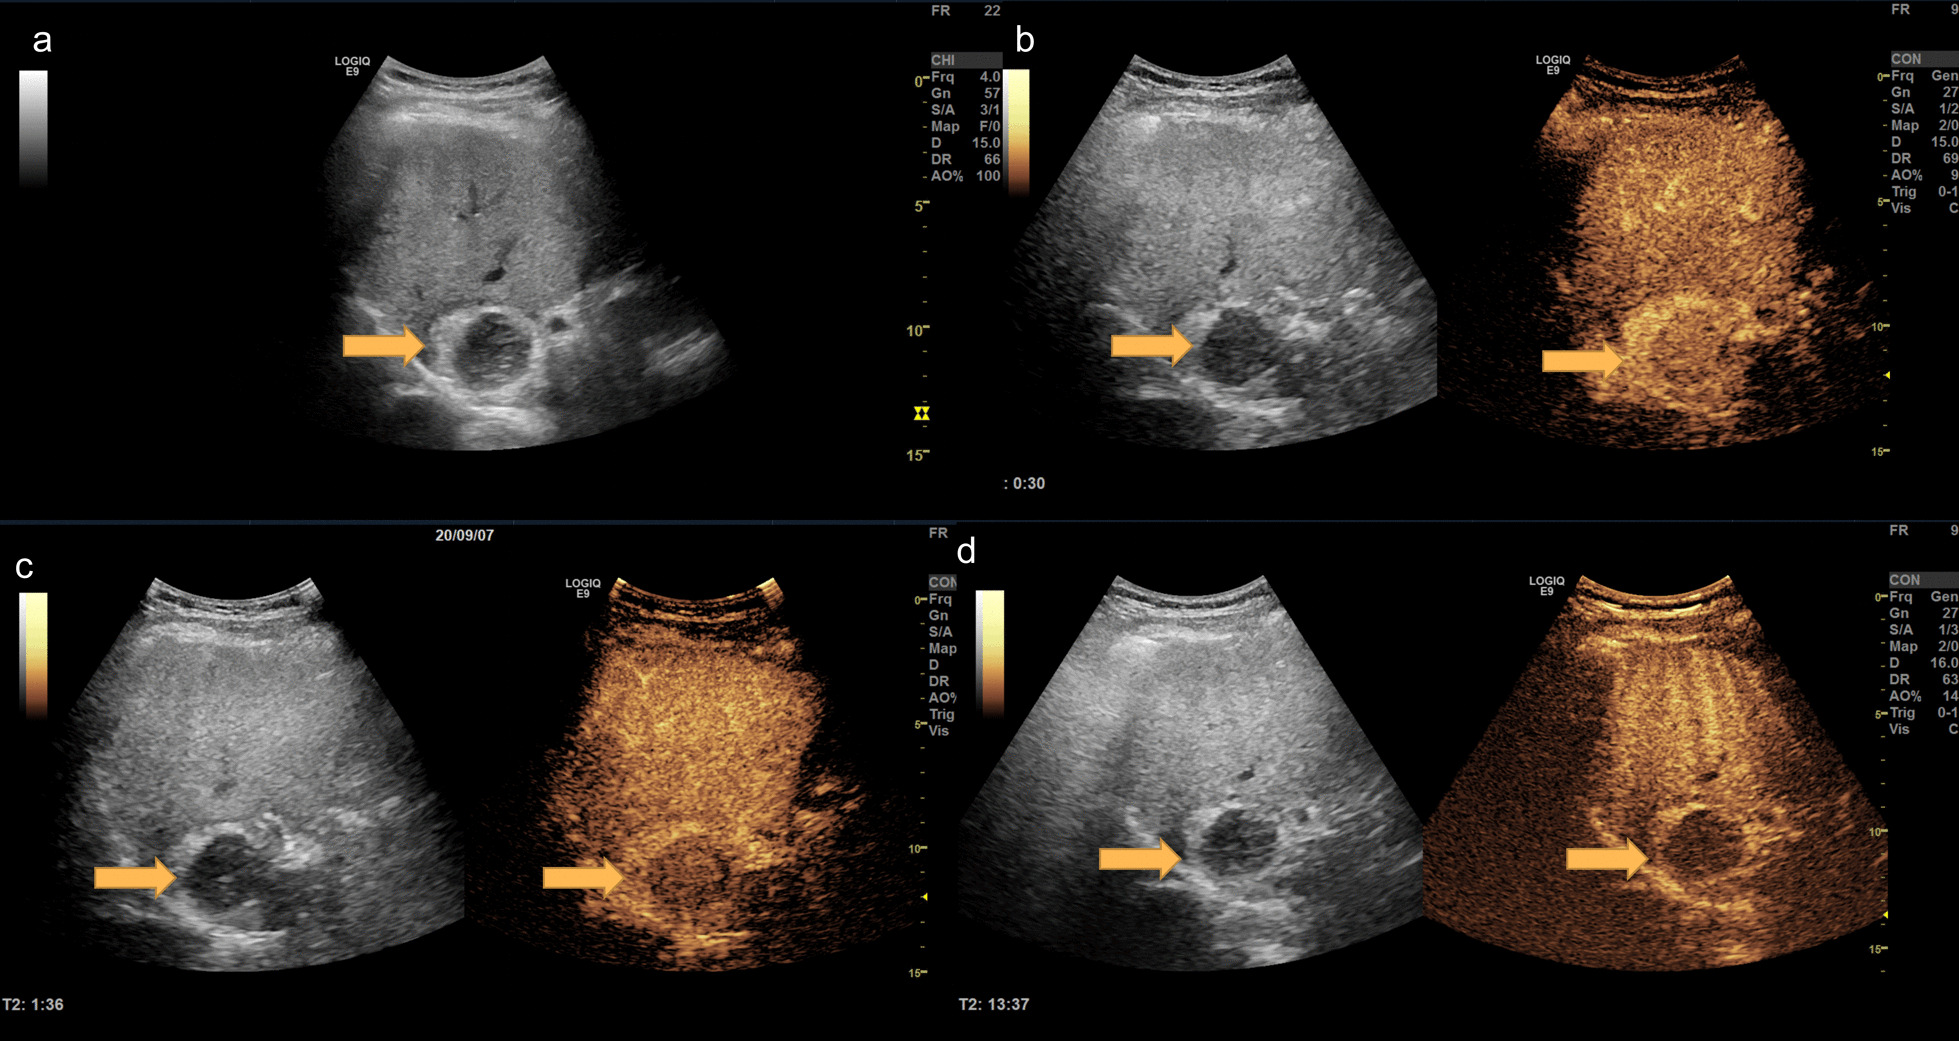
A liver mass discovered during physical examination in a 30-year-old male patient without chronic hepatitis B. The mass is estimated to measure approximately 4.6 cm × 3.6 cm and is located in the caudate lobe (a). On contrast-enhanced ultrasonography, the mass displays hyperenhancement after the injection of the contrast agent (b), contrast agent wash-out can be observed as a hypoenhancement at the center of the lesion, and the peripheral hyperechoic area remains hyperenhancing during the portal venous phase (c) and hypoenhancing during the Kupffer phase (d)
